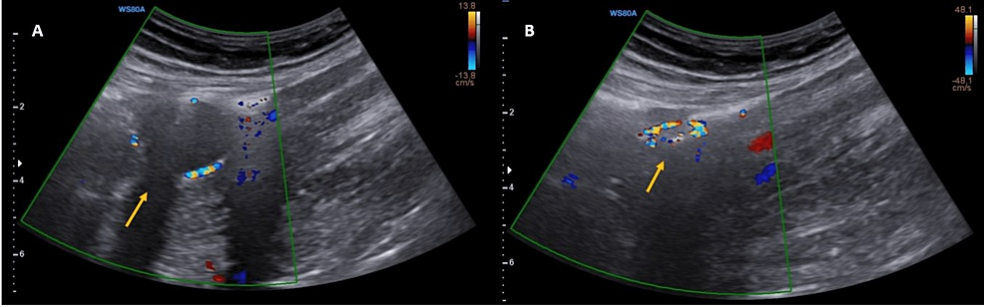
Ultrasonography of misplaced Foley catheter balloon Before (A) and after (B) deflation
